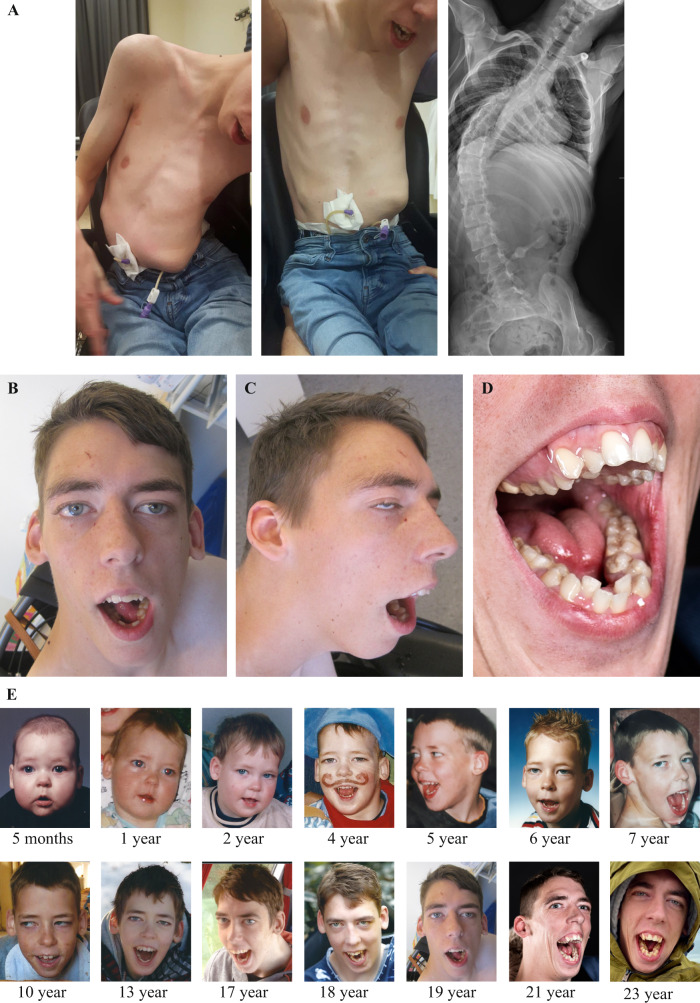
Clinical and radiology images show severe scoliosis, dysmorphisms, and dental crowding. A Clinical photographs without traction (left) and with traction (middle) showing asymmetry of the chest and rib protrusion on the left side. Radiograph of the vertebral column (right) with anteroposterior view in supine position shows a slight left convex curvature of the upper thoracic spine and severe right convex scoliosis of thoracolumbar spine (Cobb’s angle ±75°) with axial rotation (asymmetric projection of spinous processes and pedicles) and asymmetry of the thoracic cavity. B Frontal and C profile facial photographs of the proband (age 19 years), showing a long face with hypotonic appearance, long palpebral fissures, a prominent nose, and small simple ears. The photograph of the mouth (D) shows crowded teeth with gingival hyperplasia (age 21 years). Facial photographs between age 5 months and age 23 years (E), showing a progression of facial dysmorphisms with advancing age. Only mild dysmorphic features are observed in early childhood, including ptosis and a long philtrum (age 5 months to 4 years). However, the proband develops a progressively pronounced long hypotonic face with open mouth (e.g., photographs at 19 versus 10 versus 2 years of age), and has crowded teeth at age 21 (D), whereas teeth appear less crowded at earlier ages (age 7, 13, and 17 years).
